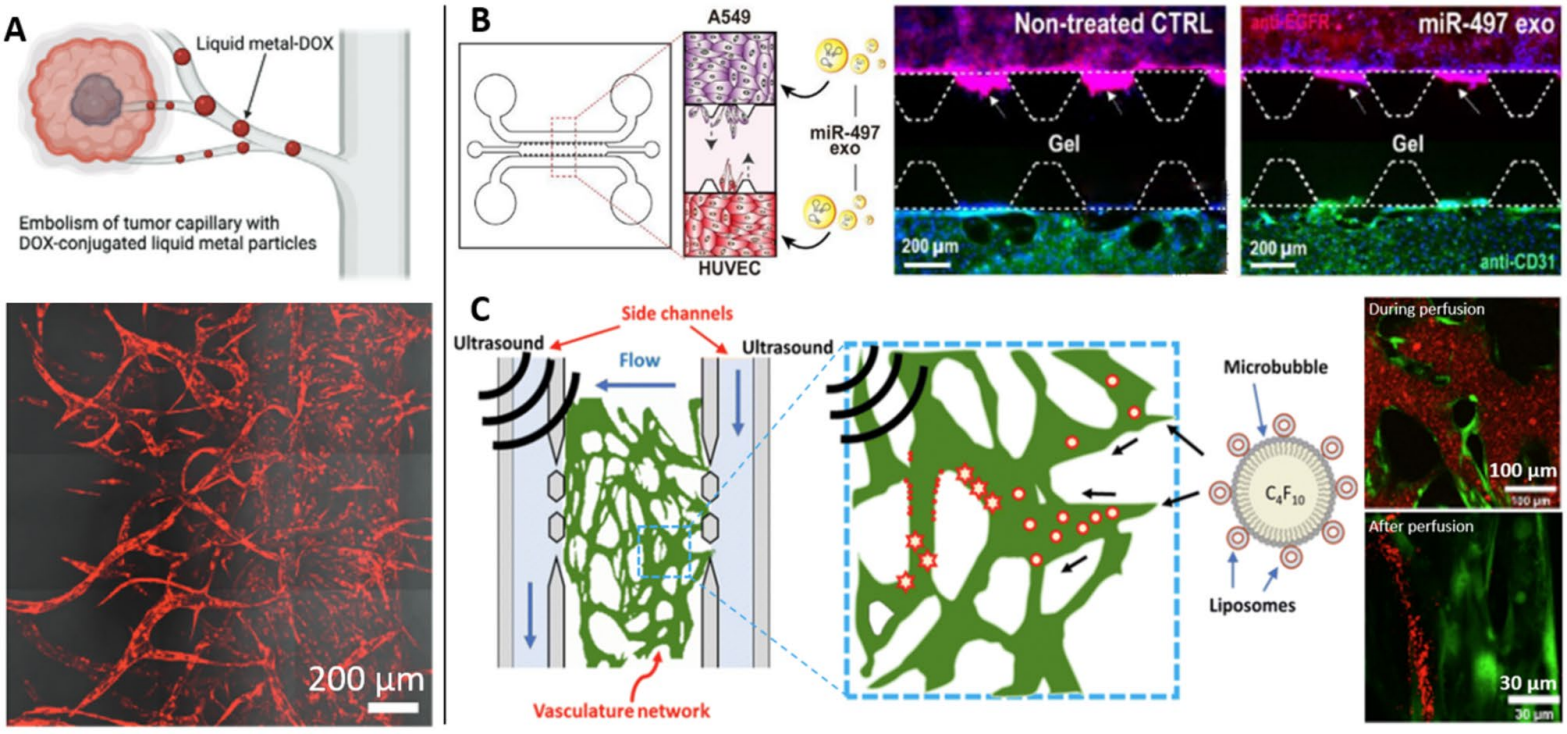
Drug delivery in VTMs. A Liquid metal NPs loaded with doxorubicin were injected within the vascularization and successfully circulated through capillaries and around the tumor in 3 days without targeting [53]. B A549 and HUVECs were seeded in side channels while collagen I was loaded in the middle. The treatment of exosomes carrying microRNA showed decreased cancer cell invasion (white arrows) as well as inhibition of the endothelial reorganization into tubular constructs [72]. C Liposomes were loaded in microbubbles that were collapsed using inertial cavitation induced by ultrasound in the vascular bed, enabling the deposition of the liposomes [112]
